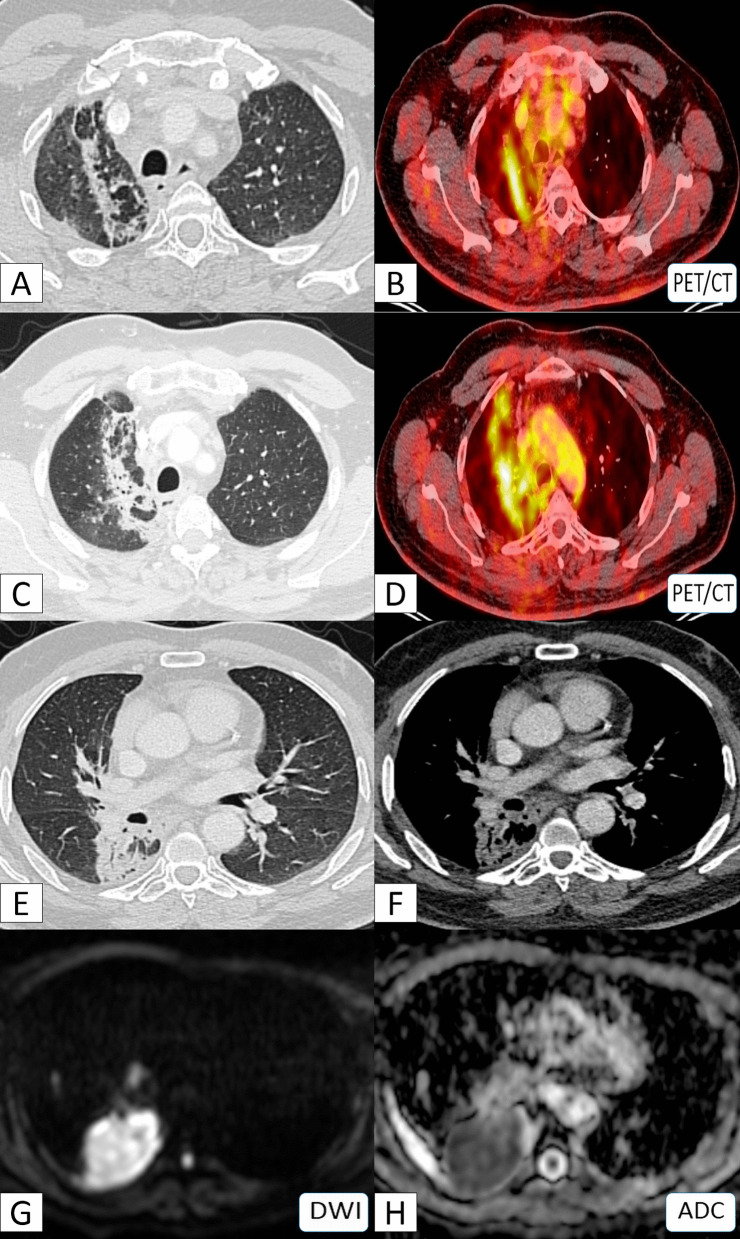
A 51-year-old male patient with a history of peripheral bronchial carcinoma in the medial aspect of the apical segment of the right lower lobe which was managed by CRT. A, C Axial chest CT (lung window) showed right apical lung scarring along the cone bead of irradiation. B, D PET/CT images showed high uptake (hot areas) at the scarring tissue. E, F Axial chest CT (lung window) showed peripheral lung carcinoma. G DWI showed diffusion restriction (bright signal) and H ADC mapping images showed corresponding low ADC value. Diagnosis: Proved patient with secondary malignancy on top of post-irradiation scarring
